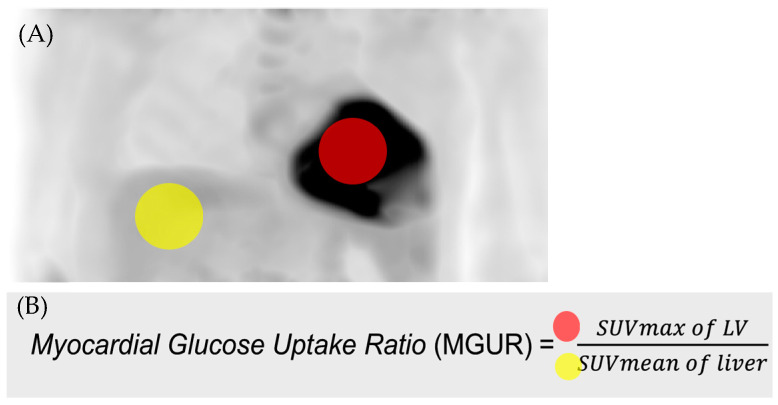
(A) 3 cm-sized spheres of Region of Interest (ROI) were drawn in the wall of the left ventricle (LV, red circle) and liver (yellow circle), respectively. (B) Myocardial glucose uptake ratio (MGUR) was calculated as SUVmax of LV divided by SUVmean of liver.
